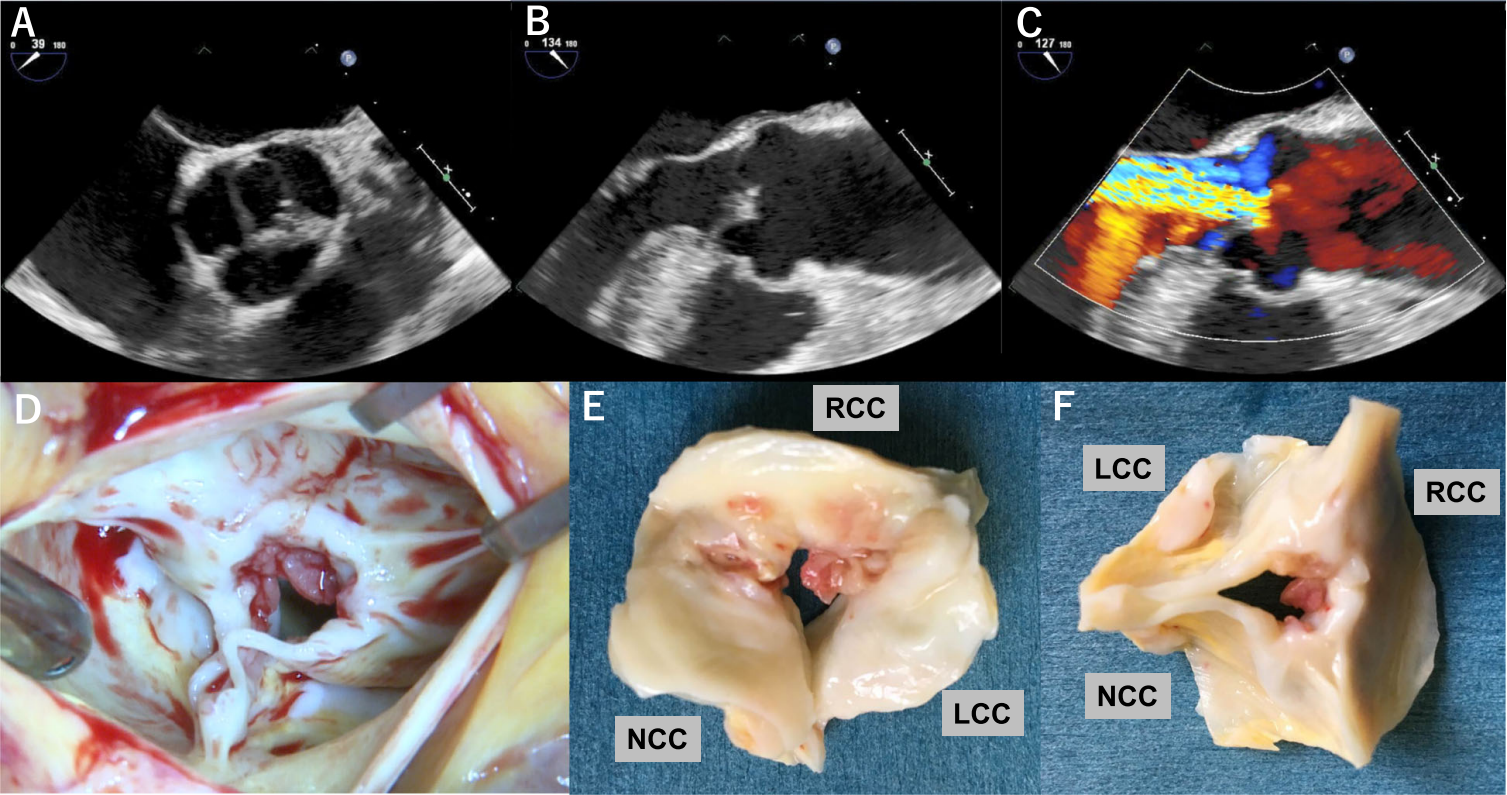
a–c Transesophageal echocardiography (a is midesophageal aortic valve short-axis view, b and c are midesophageal aortic valve long-axis view). Unicuspid aortic valve with a commissure at 12 o’clock and raphe at 4 o’clock and 8 o’clock (a). Moderate aortic regurgitant flow to posterior wall of left ventricle without any findings of valve damage, rupture of chordae tendineae and perivalvular abscess (b, c). Intraoperative finding confirmed unicuspid aortic valve with a connection of all cusps via raphe except for the commissure between left coronary cusp and noncoronary cusp, and a vegetation between left coronary cusp and right coronary cusp (d–f). *LCC* left coronary cusp, *NCC* noncoronary cusp, *RCC* right coronary cusp
